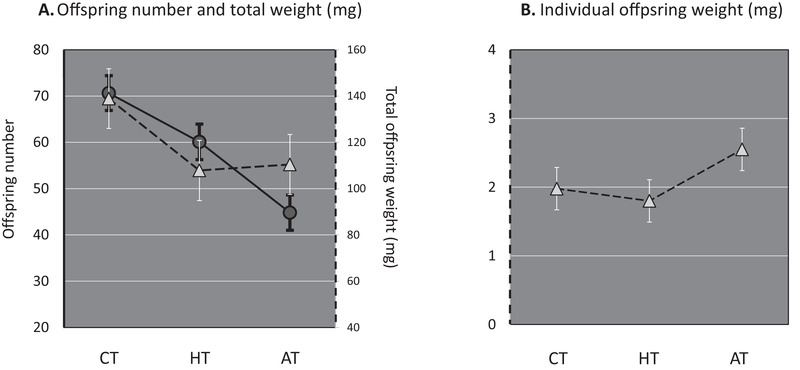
Mean fitness, total offspring weight, and individual offspring weight for each treatment. Mean fitness (adult offspring number, circles), mean total offspring weight (triangles, A), mean individual offspring weight (triangles, B), and 95% confidence intervals (linear mixed model estimates) are given for each treatment: control (CT), heterogeneous treatment (HT), and ageing treatment (AT).
